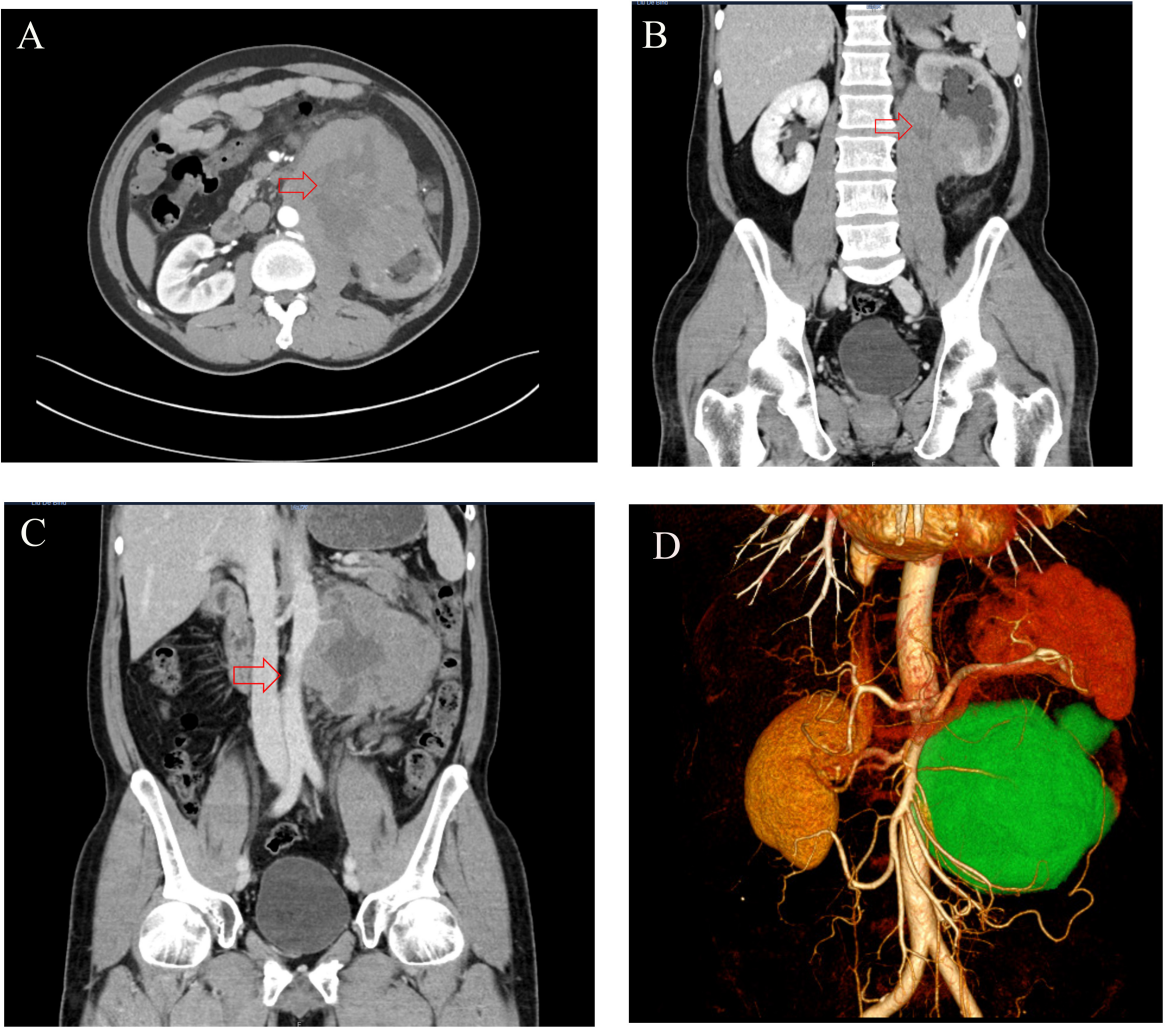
(A, B) The CT imaging revealed a large (115 × 120 × 97 mm) hypodense mass at the lower pole of the left kidney with internal heterogeneity, accompanied by left hydronephrosis and ureteral obscuration. (C, D) The CT imaging demonstrates a mass encircling the abdominal aorta and left renal artery/vein, with blood supply derived from branches of the left renal artery, superior mesenteric artery, and inferior mesenteric artery (in panel D, the tumor is labeled in green, the kidney is in brown, and arteries are in red).
